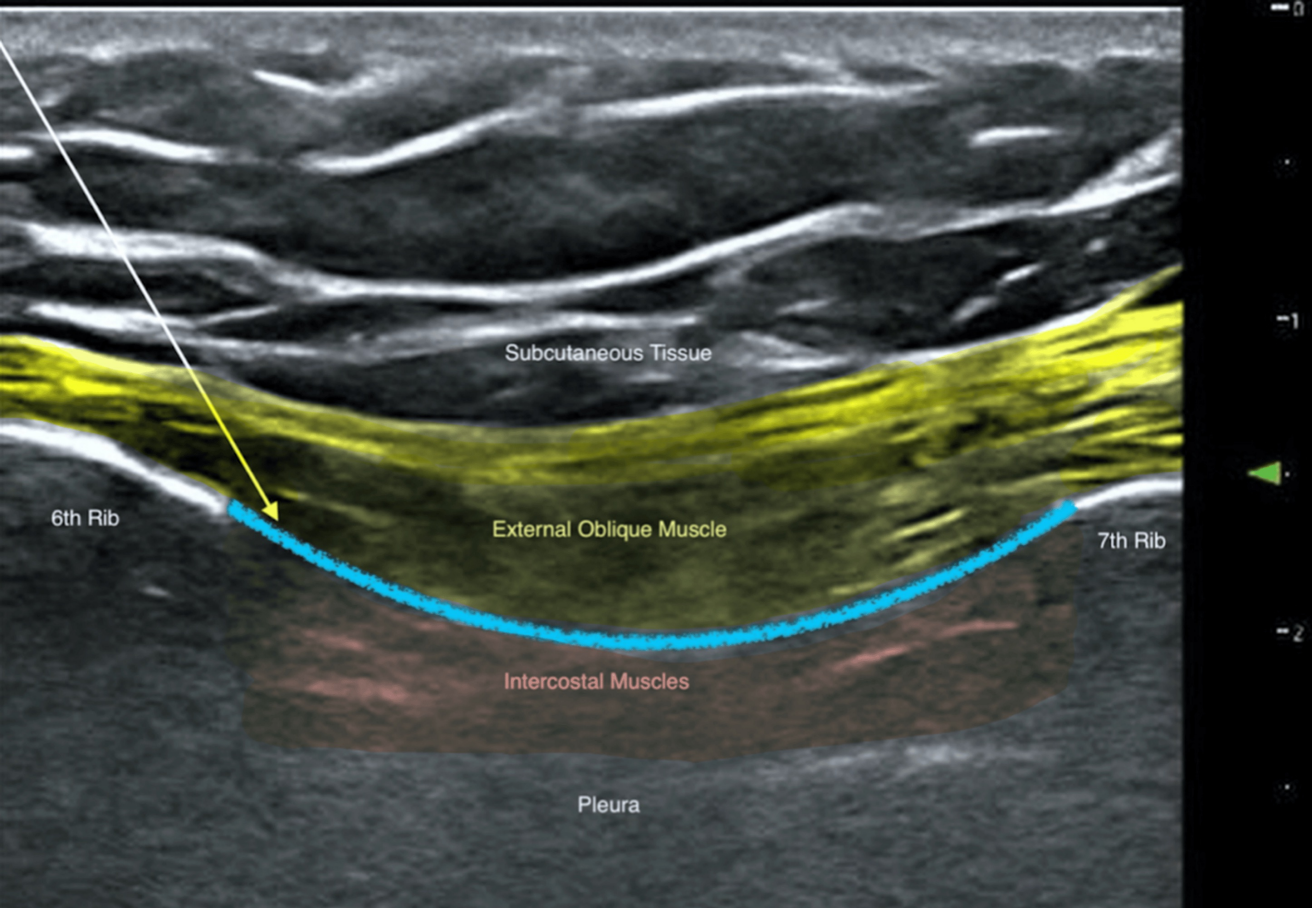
An ultrasound image of the EOI block A linear ultrasound probe was placed in the sagittal oblique plane, with the probe's cranial end rotated slightly medially, at the level of the sixth rib, between the mid-clavicular and anterior axillary lines. The surrounding structures, including the sixth and seventh ribs, subcutaneous tissue, external oblique muscle, and pleura, are labeled. The white arrow indicates the path of the block needle. The yellow highlight outlines the external oblique muscle. The red highlight outlines the intercostal muscles. The blue highlight denotes the fascial plane target for the EOI block. EOI: external oblique intercostal
